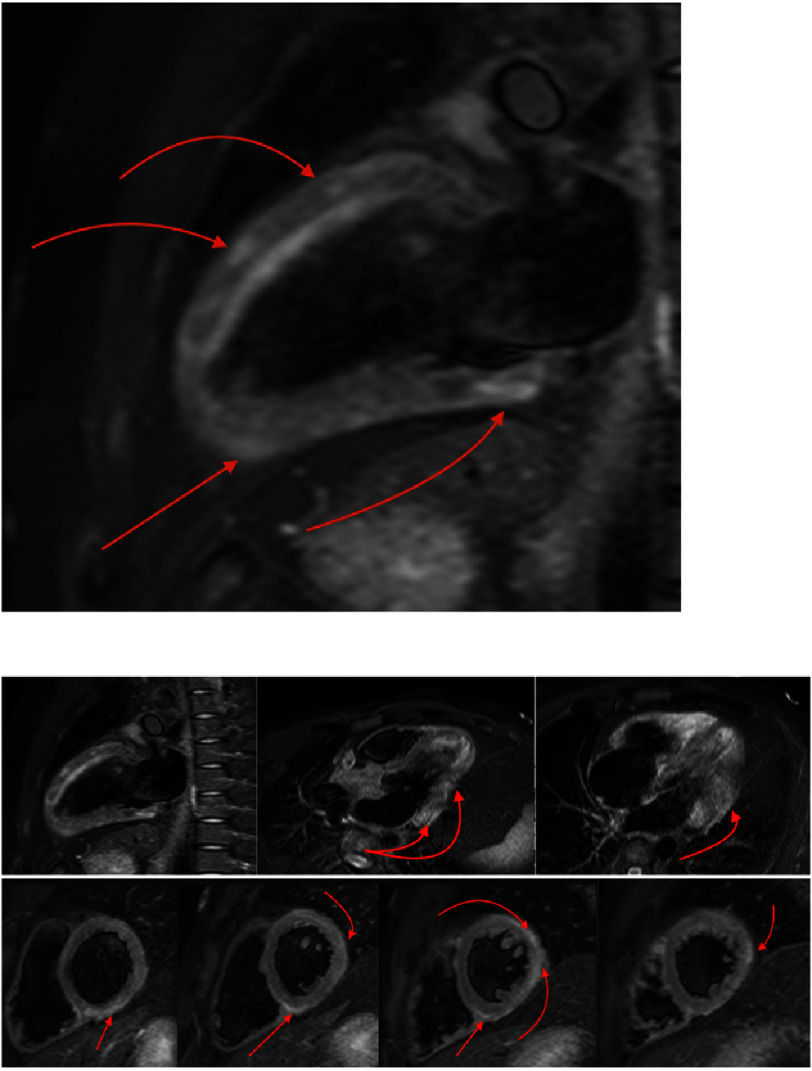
T2-weighted STIR images demonstrating myocardial edema and inflammation.
